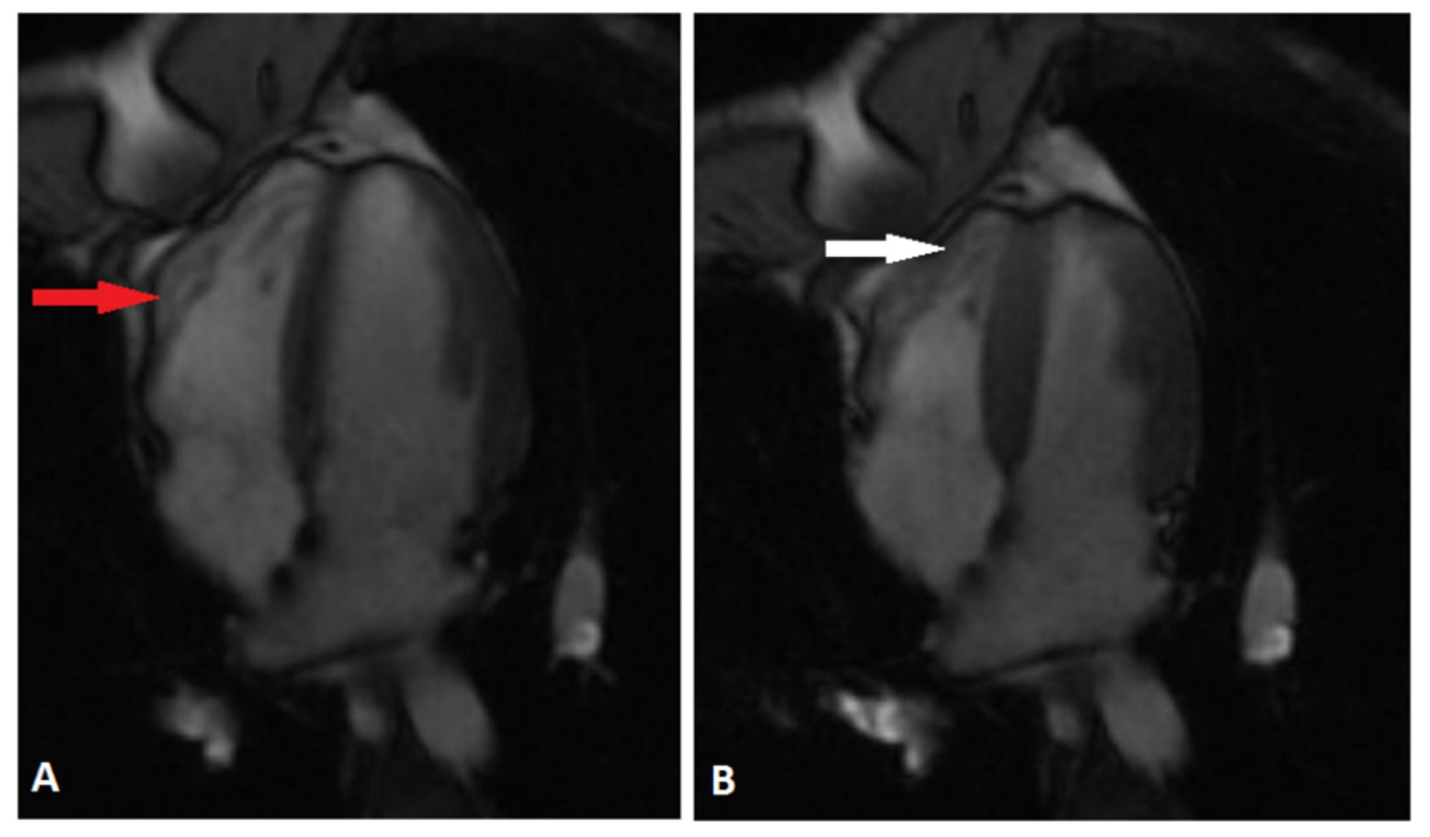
Magnetic resonance imaging (MRI) of heart with and without contrast showing aneurysmal dilatation of right ventricular free wall (red arrow) and focal outpouchings of the right ventricular free wall (white arrow); A-Diastole, B-Systole
